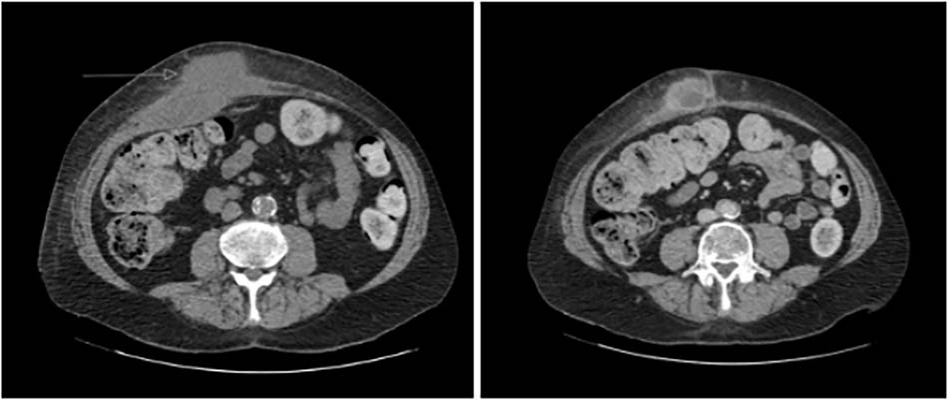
CT abdomen with portal venous contrast in axial plane showing extension of collection towards the paraumbilical region.
